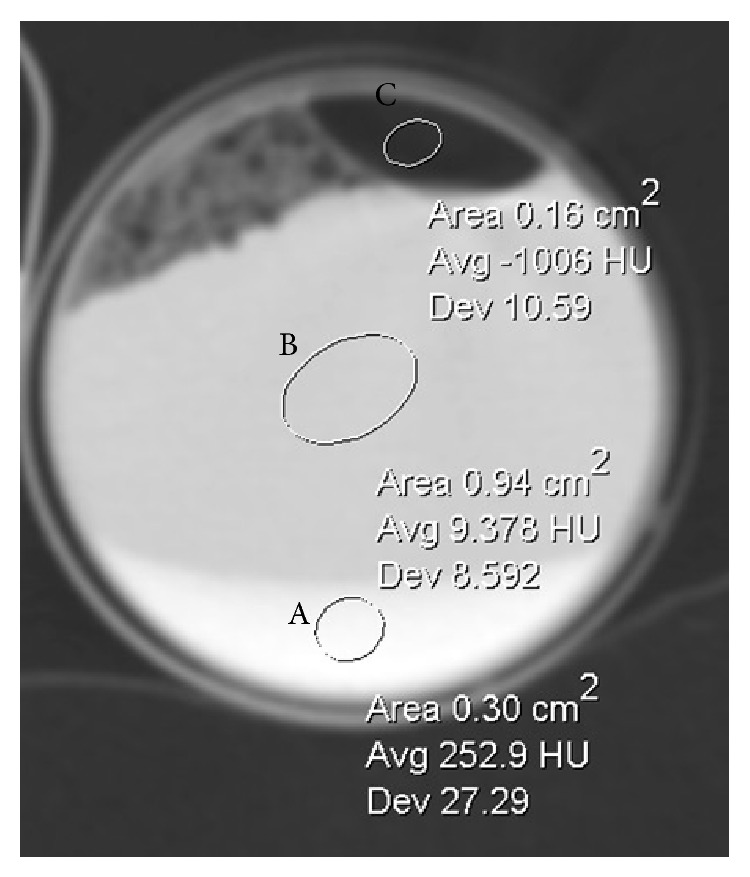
High-attenuation of talc. CT image of a syringe of saline mixed with talc demonstrates high density sediment of the talc (A) and fluid density of the supernatant saline (B). Note also the gas at the top (C).
